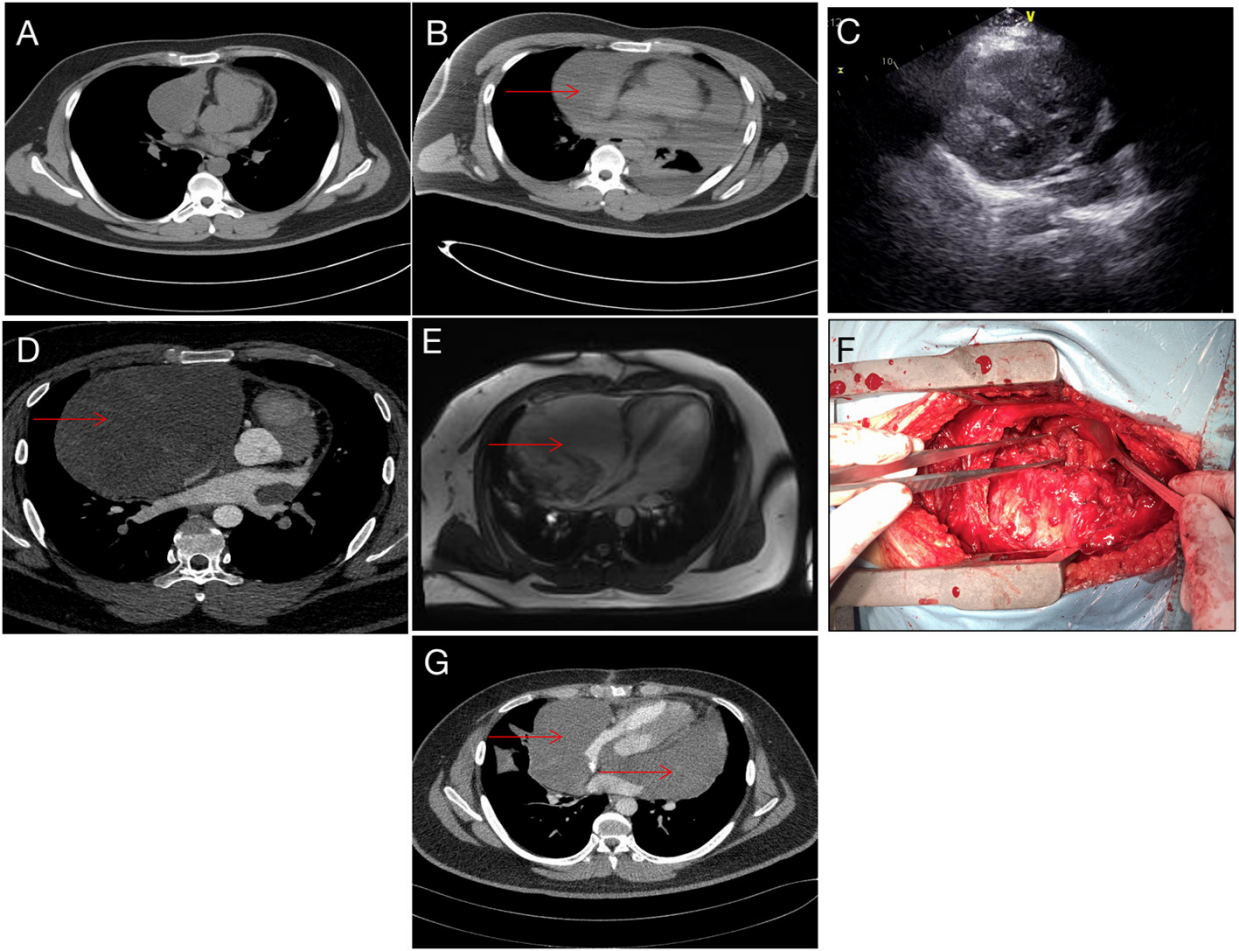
Imaging data. (A) CT image taken during the first admission. (B) After pericardiocentesis, CT indicating a suspicious mass (red arrow). (C) Echocardiography showing pericardial space-occupying lesions. (D) Reexamination after 5 months showed obvious space occupation (red arrow). (E) Preoperative magnetic resonance imaging, right pericardium, showing a cystic mass (red arrow). (F) During the operation, the tumor filled the pericardial cavity. (G) Tumor recurrence noted 5 months after operation (red arrow).
